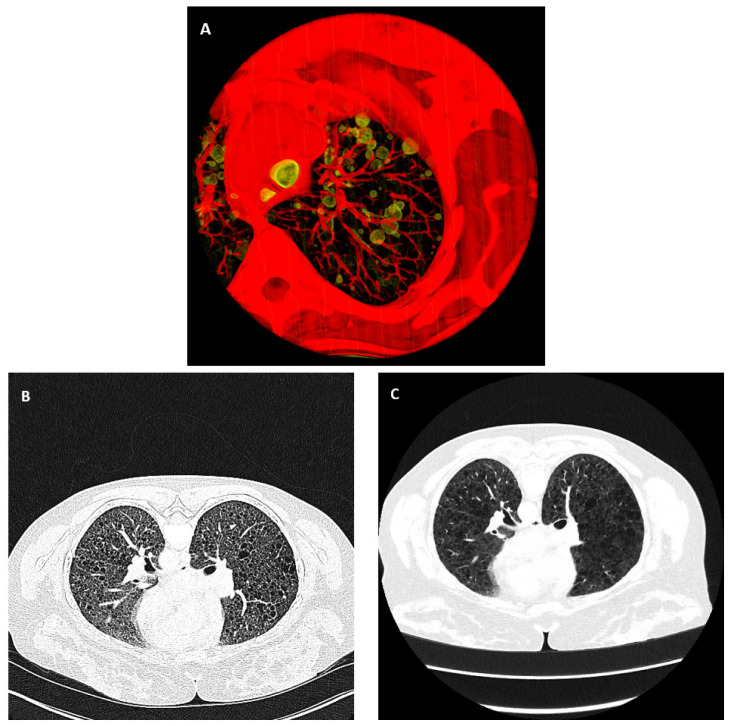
Air-filled pulmonary cysts from the disease lymphangioleiomyomatosis and the variability of chest CT images over time and across different scanner platforms. (A) A three-dimensional rendered view of a portion of the lung from the chest CT scan of a patient. The bubble-like structures in the lung highlighted in yellow are air-filled cysts, a hallmark of the disease. (B,C) High-resolution CT images of the same location in the chest of the same patient were acquired 12 years apart from two different scanner platforms. Both are displayed in the grayscale range of −1100 HU to 0 HU. This is an example of the variability of image quality and characteristics.
